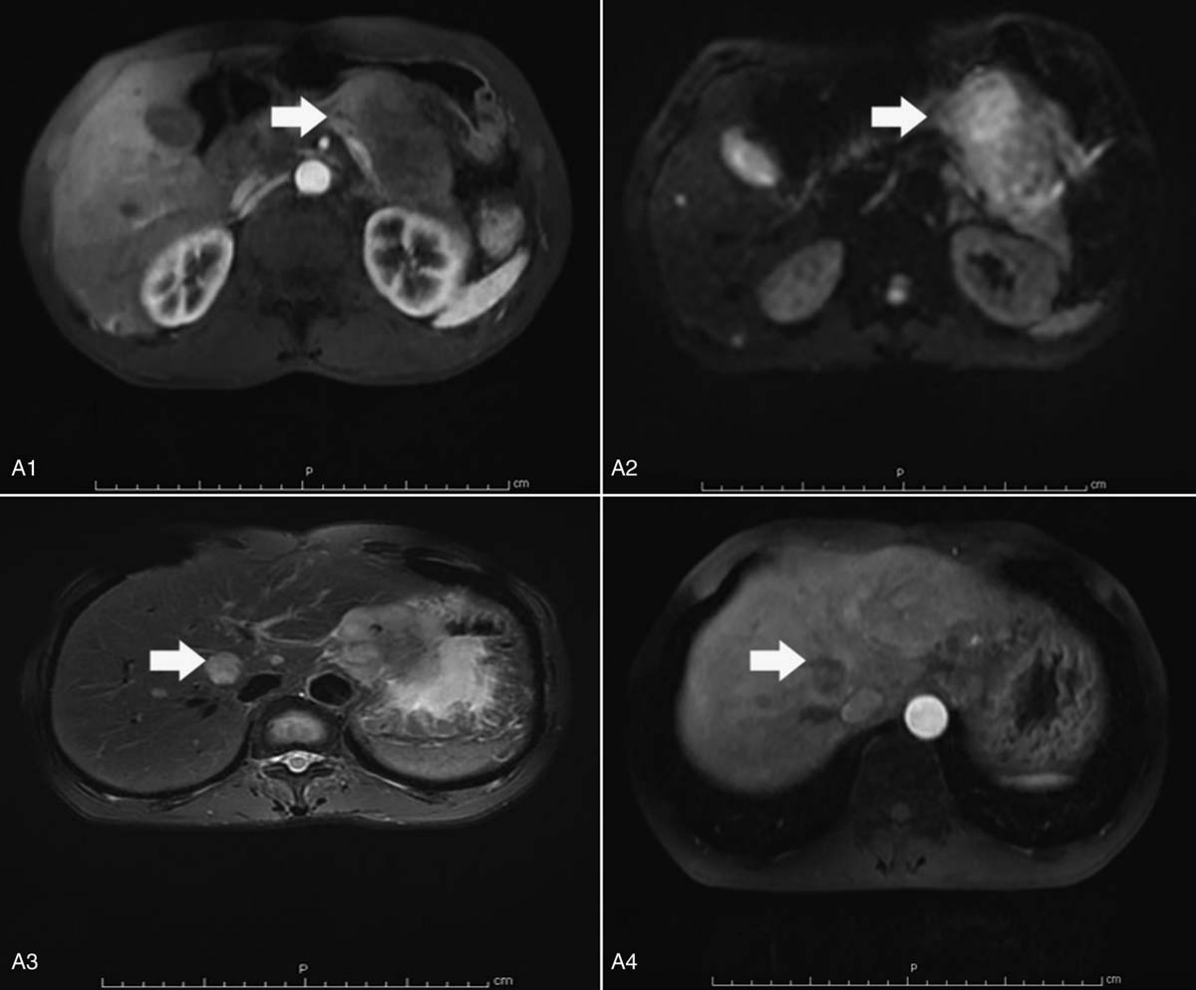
Radiological examination of the reported case. Abdominal MRI scanning showed a hypoattenuating lesion located in the body and tail of the pancreas, invading the spleen vein. MRI = magnetic resonance imaging.
